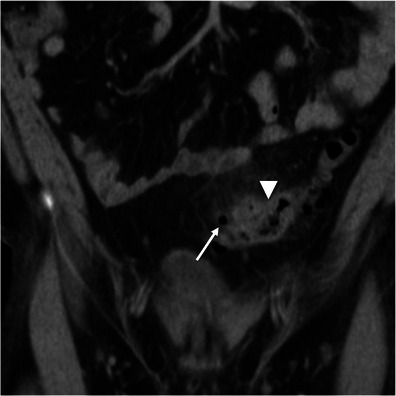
Acute diverticulitis. Reformatted coronal contrast-enhanced CT scan showing sigmoid diverticuli (*arrow*), mild bowel wall thickening (*arrowhead*) and pericolonic disproportionate fat stranding. These findings are compatible with sigmoid diverticulitis
